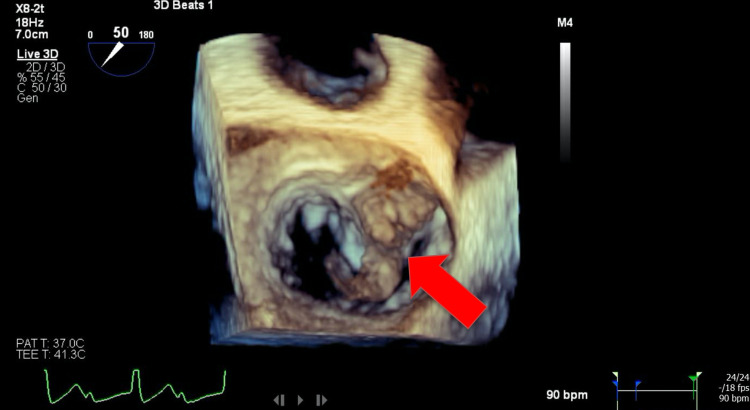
Transesophageal echocardiogram 3D image showing anterior mitral leaflet vegetation (red arrow)
